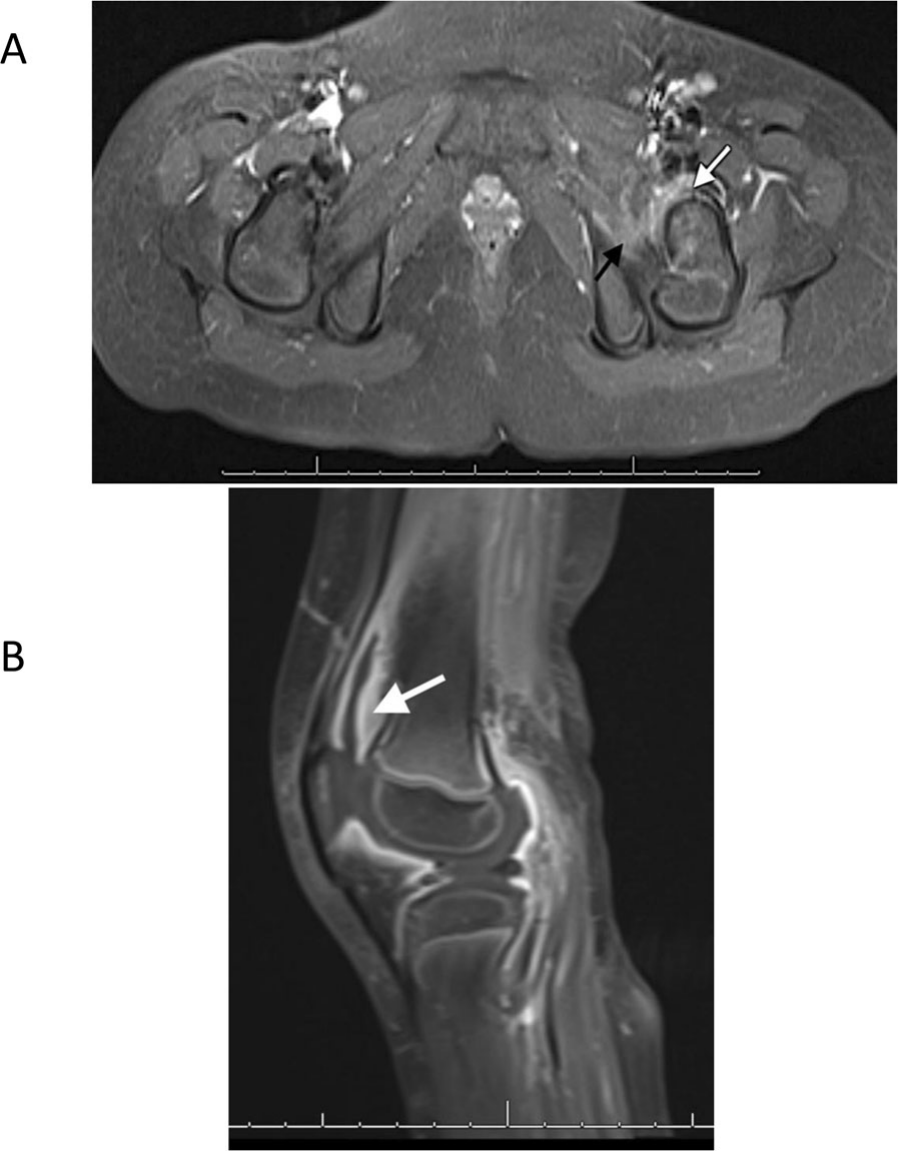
Radiographic findings of reactive arthritis following Hia sepsis. a, Left-sided joint effusion with synovial enhancement noted in the left hip (white arrow). In addition, asymmetric myositis involving the lateral fibers of the left gluteus medius/minimus and the proximal/deep fibers of the vastus intermedius and vastus lateralis musculature is also observed (black arrow) b, Enhancement and soft tissue swelling was noted by MRI of left knee with synovial thickening (white arrow) and effusion present
